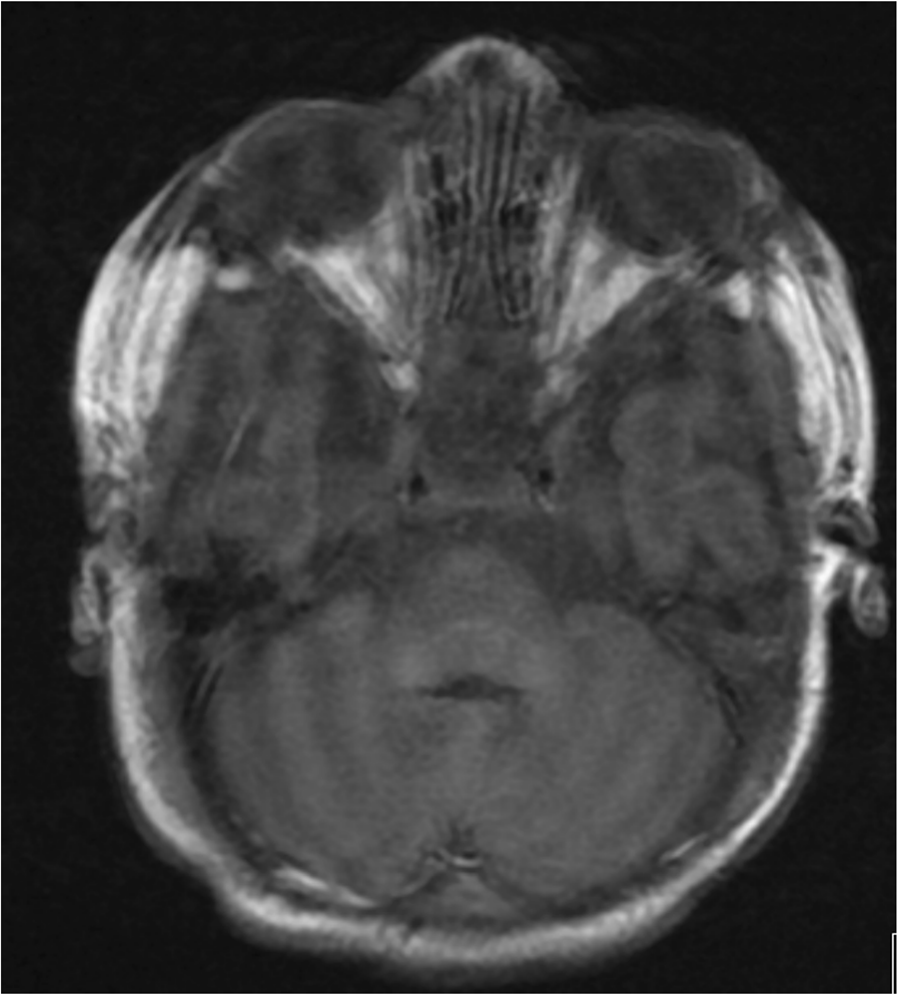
MRI T1 axial scan showing a bilateral bleeding along the cerebellar convexity
